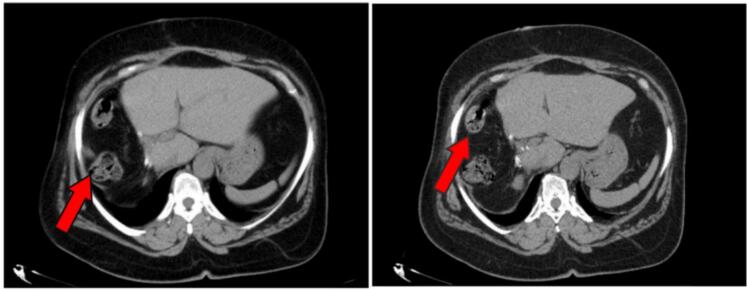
CT images of control objectifying the absence of local recurrence, presence of some a few bowel in the hepatectomy compartment indicated by the red arrow. (For interpretation of the references to colour in this figure legend, the reader is referred to the web version of this article.)
